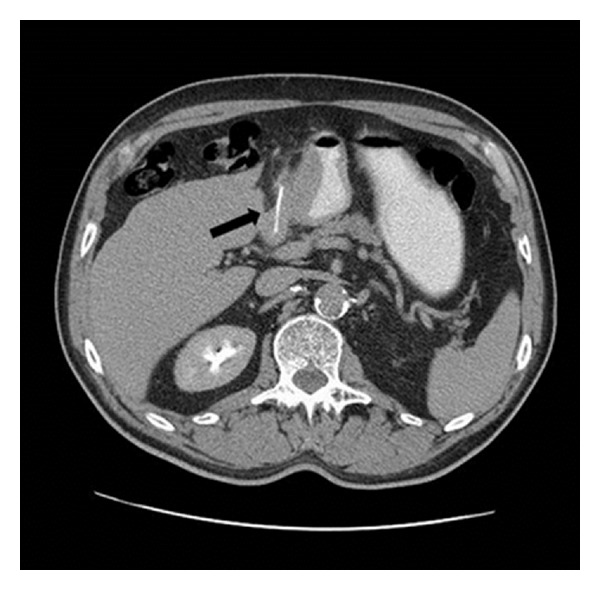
CT scan: submucosal heterogeneous enhancing mass in the distal stomach, that produced a stricture of the gastric lumen. *Black arrow* shows the presence of linear radiopaque foreign body.
